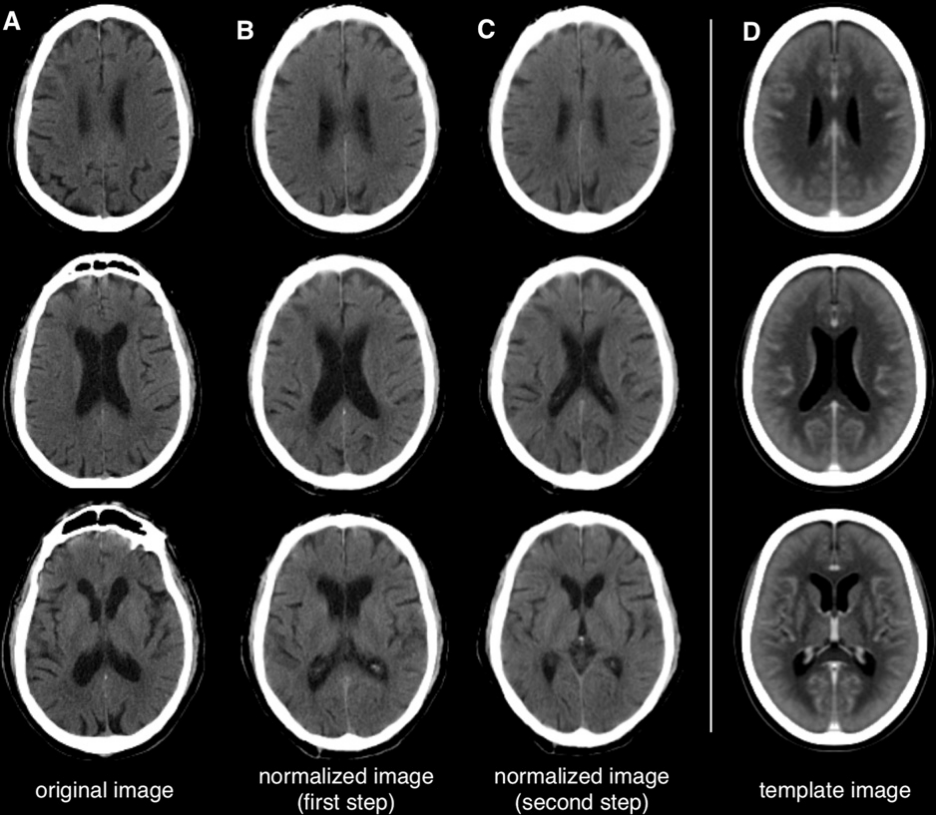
Spatial normalization of CT images to MNI space. A representative brain CT image, shown in three axial views. Section (A) is warped to MNI space in two steps (B–C), using a template CT image (D) as reference image. Note that the first normalization step registers the brain contour to the template, whereas the second step primarily registers periventricular regions.
